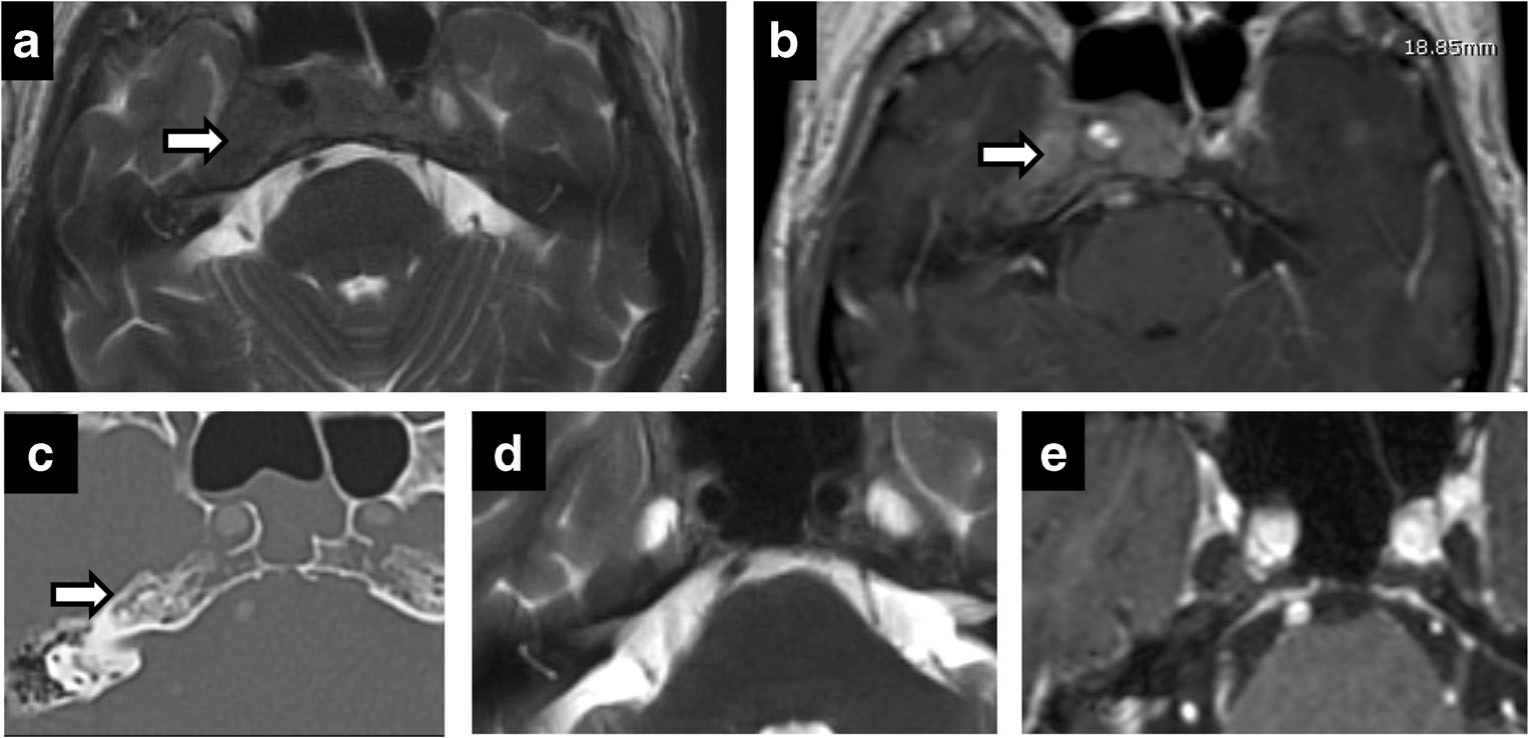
Lymphoma. Axial T2 (a), axial C+ (b), CT (c). Bilateral CN VI palsy and right facial pain. Mottled appearance of clivus and petrous apices on CT—caused by T2 hypointense, enhancing infiltrating lesion. Post treatment (d, e)—complete resolution
